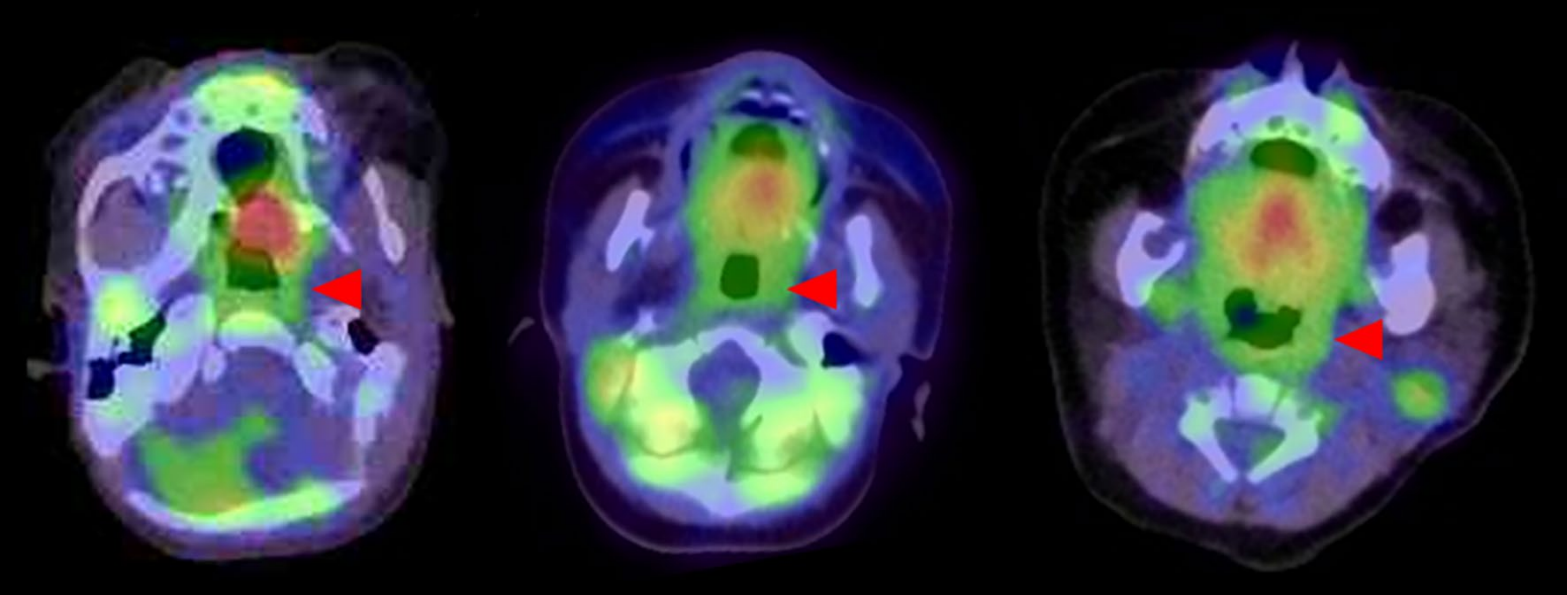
FDG PET/CT image of tonsil from neonates to infants. a 15 days after birth, b 38 days after birth, c 61 days after birth. Physiological intense FDG uptake in the and tonsils (red arrowhead) appears to be less frequent in the neonate and infants
